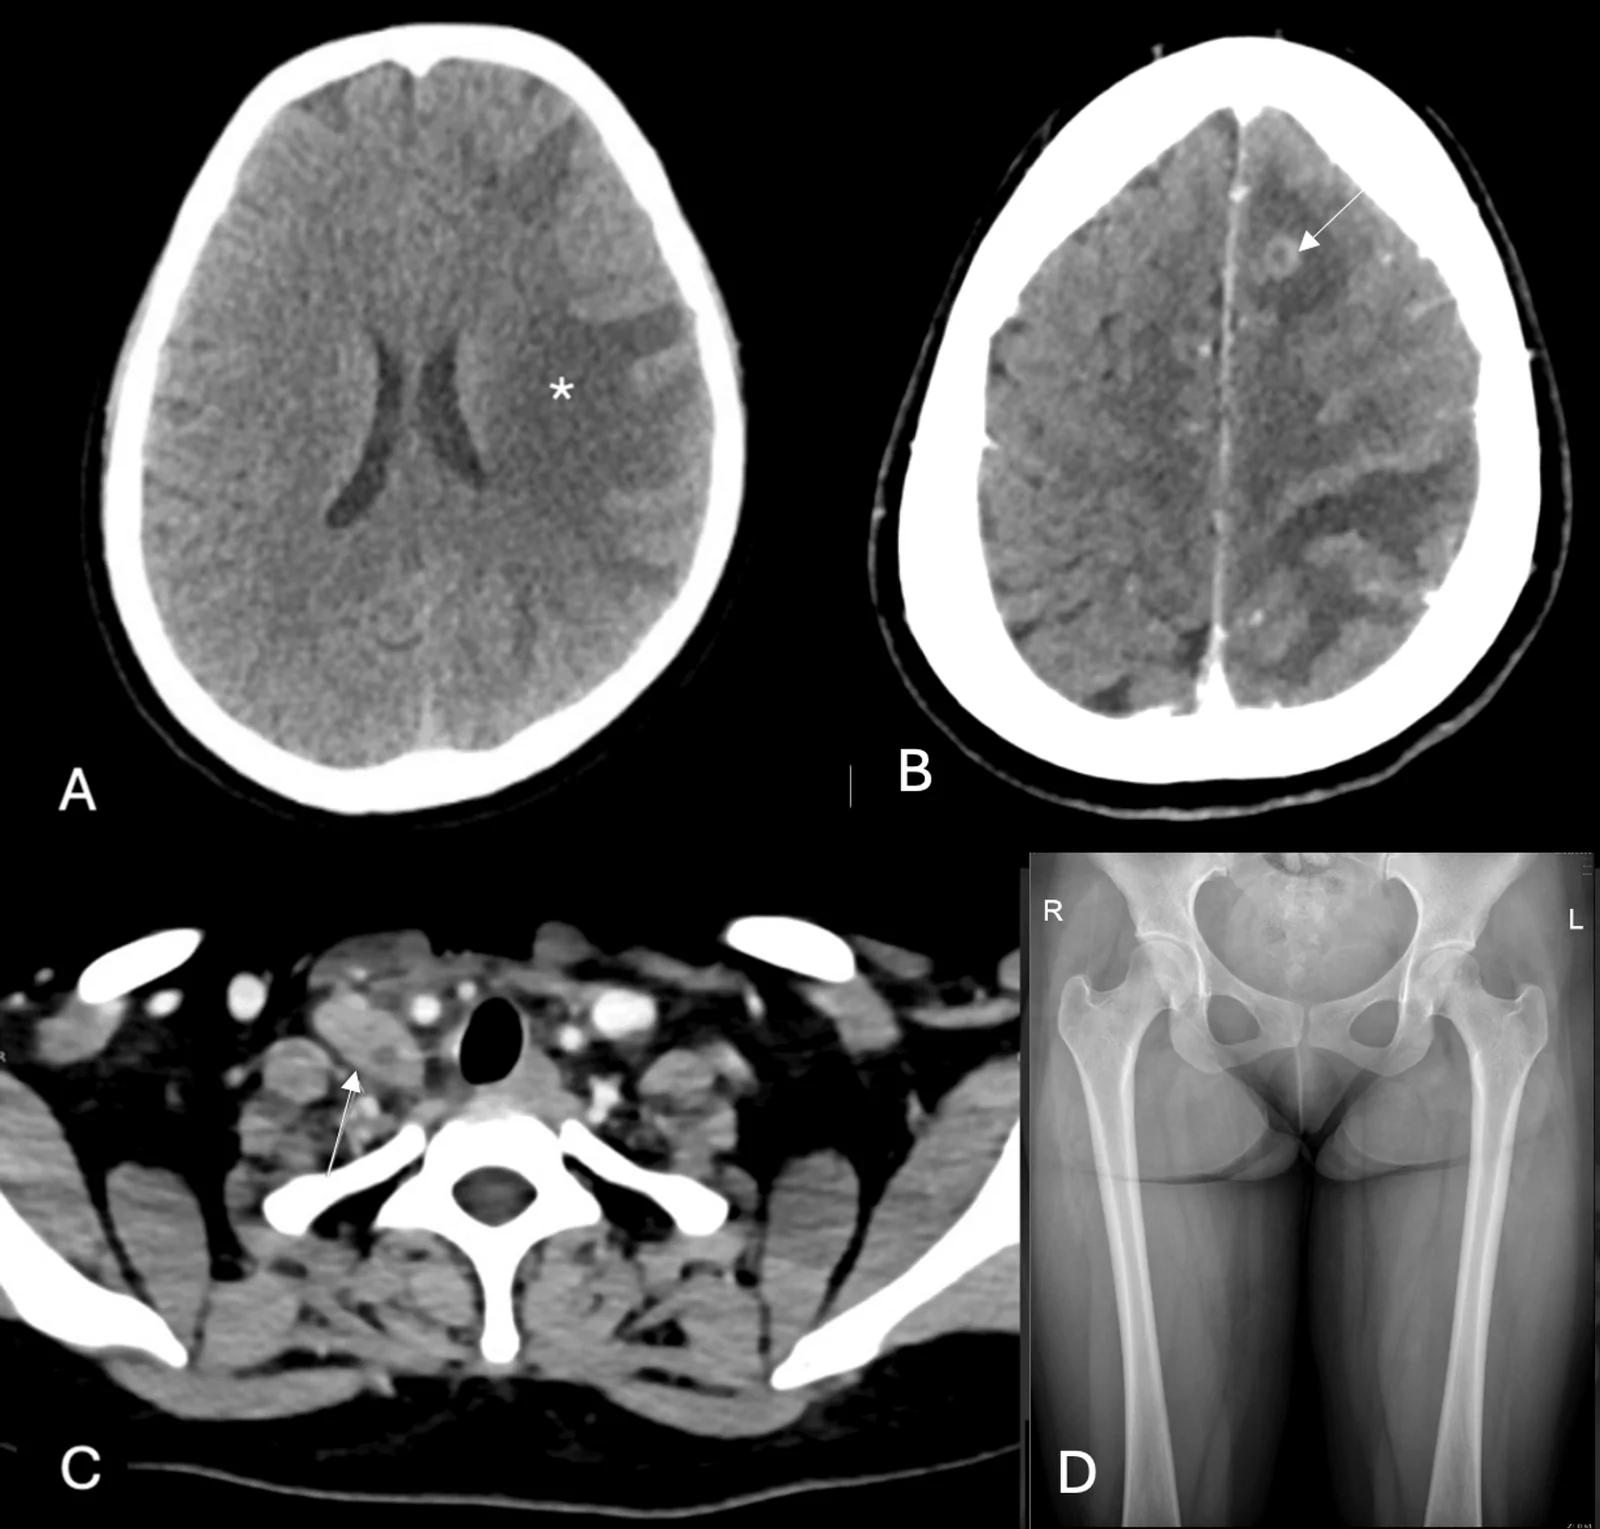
Initial imaging findings. (A) Non-contrast CT head demonstrates a large area of hypoattenuation (asterisk) in the left frontoparietotemporal region. (B) Contrast-enhanced CT of the brain shows multiple well-defined ring-enhancing lesions (arrow) in the left cerebral hemisphere with surrounding vasogenic edema and sulcal effacement. (C) Contrast-enhanced CT of the neck demonstrates necrotic right superior mediastinal (paratracheal) lymphadenopathy (arrow). (D) Skeletal survey of the extremities demonstrates no evidence of calcified intramuscular cysticerci suggestive of neurocysticercosis.
